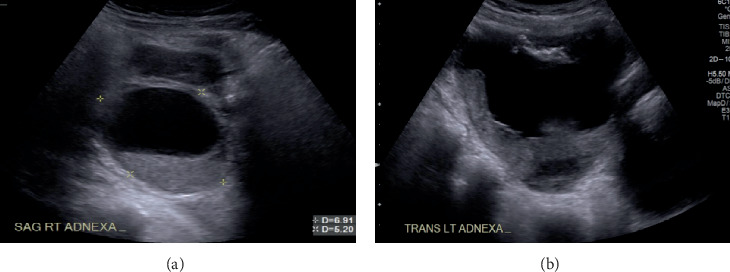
Pelvic ultrasound. (a) There is a mixed cystic and solid mass with irregular mural nodules and papillary projections measuring approximately 6.4 × 5.7 × 5.5 cm in the right adnexa. (b) There is another mixed cystic and solid mass with irregular mural nodules and papillary projections measuring approximately 8.8 × 11.4 × 8.5 cm in the left adnexa.
